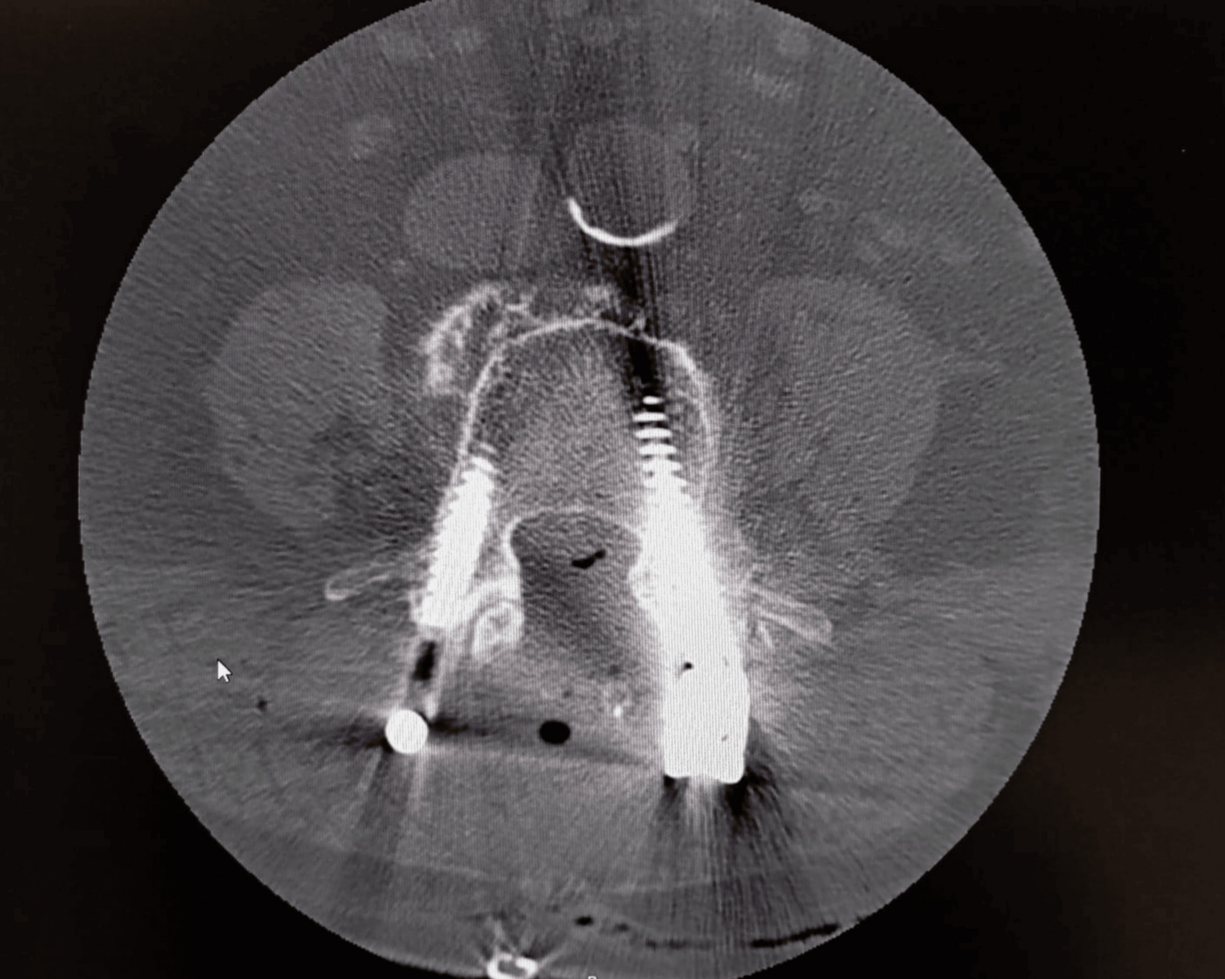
Postoperative spinal CT scan Cross-sectional (axial) CT scan of the lumbar spine with the two screws visible on either side of the vertebra, inserted bilaterally into the pedicles, suggesting proper positioning.
